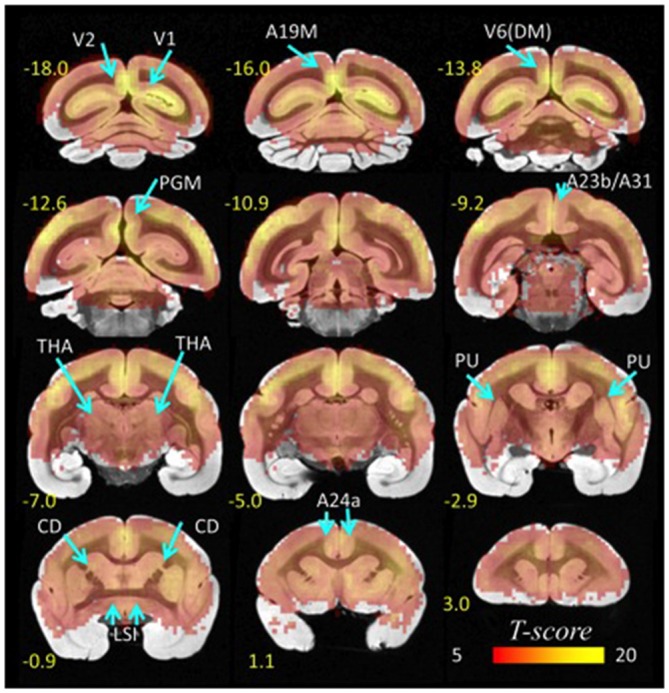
Statistical significance of *l*FCD superimposed on coronal T2 MRI views of the marmoset brain. The most prominent *l*FCD hubs were located in V1 and V2, the primary and secondary visual areas; A19M, the medial part of cortical area 19; V6(DM), the DM, dorsomedial part of visual area 6 (V6); PGM, the medial part of parietal area; PG, posterior cingulate areas A23b and A31, THA, thalamus; PU, putamen and CD, caudate; LSI, the intermediate part of the lateral septal nucleus; and cingulate area A24a. Statistical model: within-subjects ANOVA; statistical threshold: P_FWE_ < 0.05.
